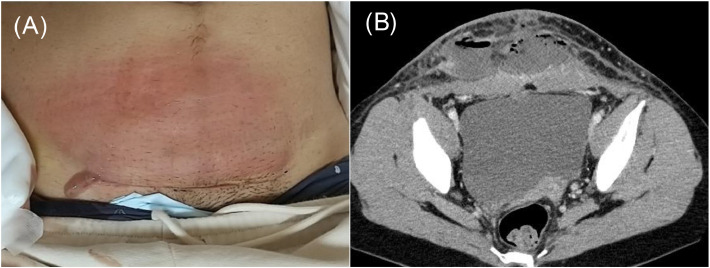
(A) Abdominal findings: the erythema, warmth, and tenderness noted in a band around the head of the wound in the lower abdomen and pus discharge from the right end of the wound. (B) Contrast-enhanced computed tomography scan revealing a fluid collection with foamy gas formation extending from both rectus abdominis muscles to the subcutaneous tissue. The lesion was encapsulated by an enhancing rim and surrounded by fat strands, suggestive of abscess formation.
